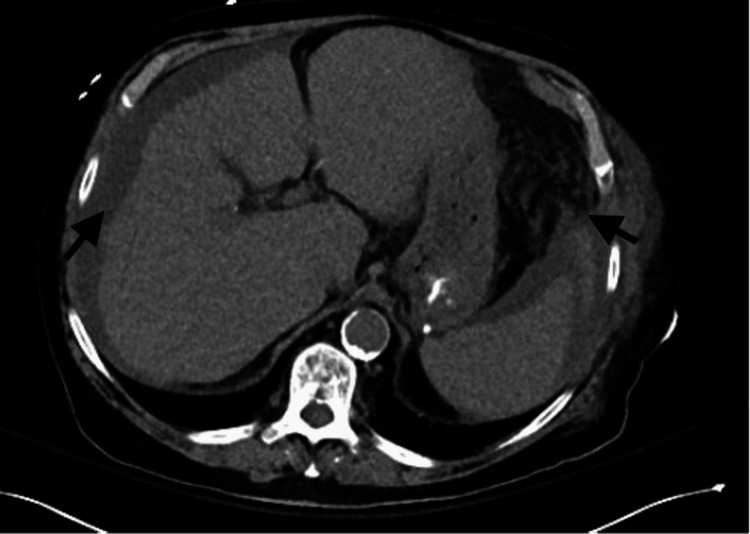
CT abdomen and pelvis: mild ascites indicated by black arrow (right). Left-sided flank fat stranding indicated by black arrow (left)
